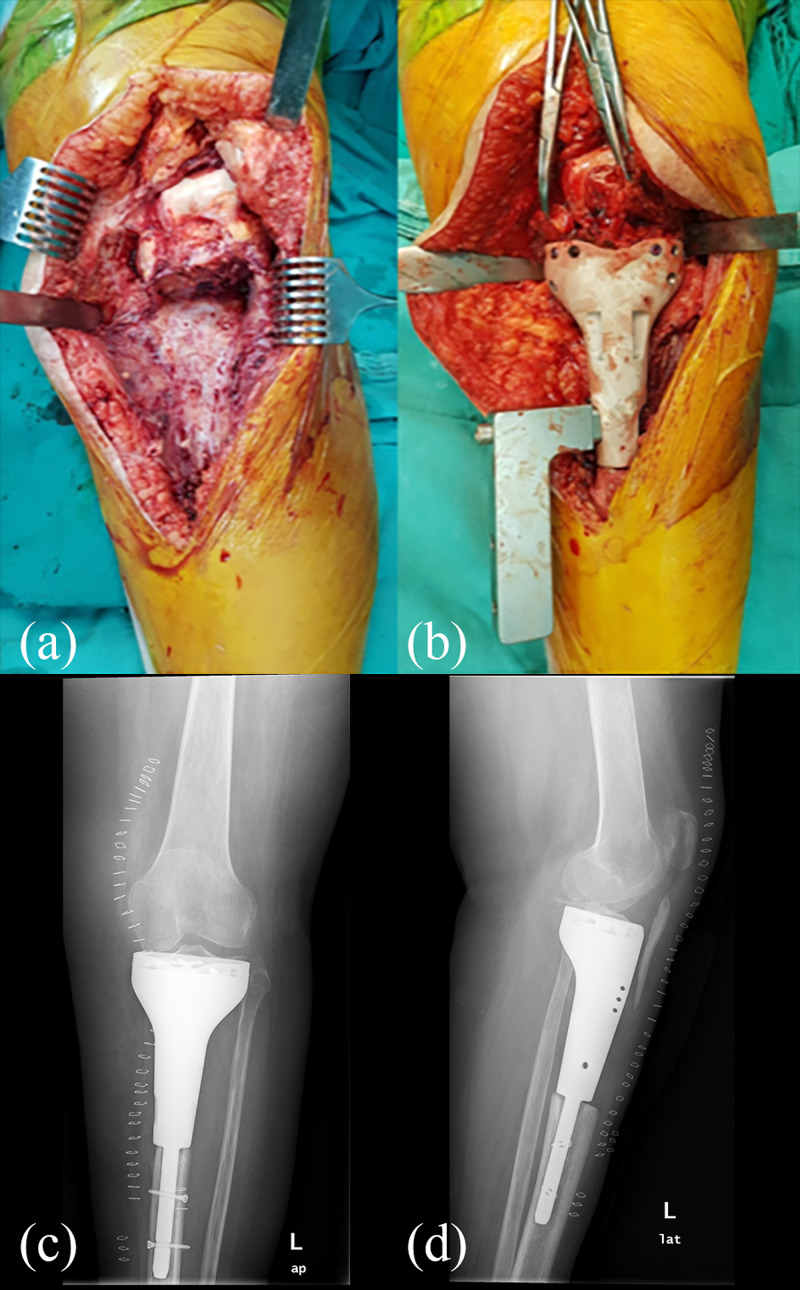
Perioperative images and postoperative X-rays (a,b) During the surgery, careful dissection of the surrounding soft tissues was ensured; the images show the implantation stage of the reconstruction; (c,d) early postoperative X-ray images
